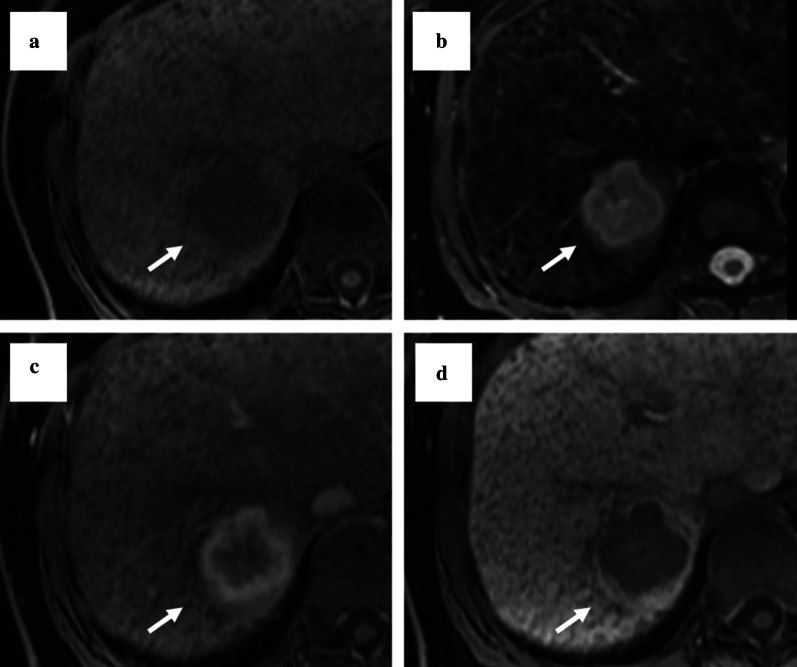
Findings of magnetic resonance imaging (MRI). a The tumor shows a low signal intensity on a T1-weighted image. b The tumor shows a high signal intensity on a T2-weighted image. c At the early phase on ethoxybenzyl magnetic resonance imaging (EOB-MRI), the tumor shows ring-like enhancement. d The enhancement is not detected throughout the tumor in the hepatocyte phase on EOB-MRI
